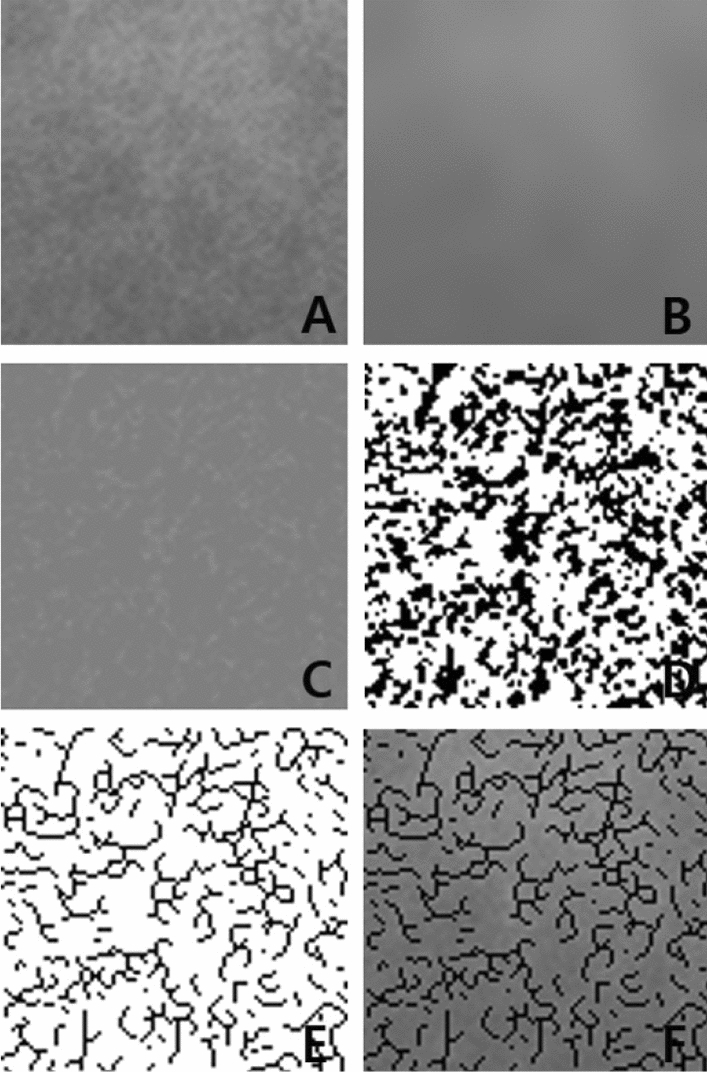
Digital analysis of the trabecular bone morphology. (A) A region of interest of the trabecular bone located in the center of the osteosarcoma (OS) area on the left posterior mandible in a patient’s panoramic radiograph in Fig. 1. (B) Result after blurring this region. (C) Result after subtracting (B) from (A) and adding 128 (D) binary versions of the image (C). (E) The trabecular pattern is skeletonized. (F) Addition of images (A) and (E) to visually demonstrate that the skeletonized image corresponds to the original trabeculae.
